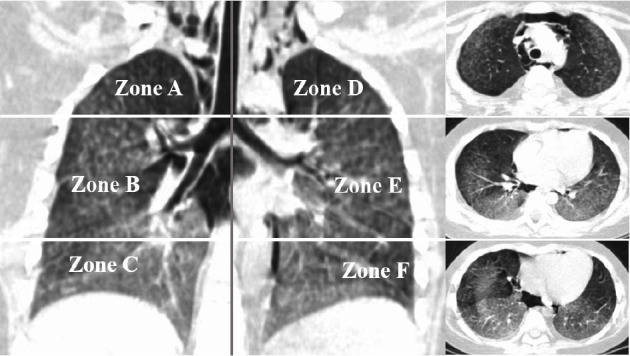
A 9-year-old girl of JDM-ILD. The KL-6 value was 3949 U/ml. The level of pulmonary vein and 1 cm above carina divide the lungs into upper, middle, and lower parts. The right lung was marked from top to bottom as Zone A to C. The left lung was marked from top to bottom as Zone D to F. The score of each zone was equal to image pattern points multiplied by distribution range points. Zone A score = 3 × 2, Zone B score = 3 × 3, Zone C score = 3 × 3, Zone D score = 3 × 2, Zone E score = 3 × 2, and Zone F score = 3 × 2. Total CT score = 42.
